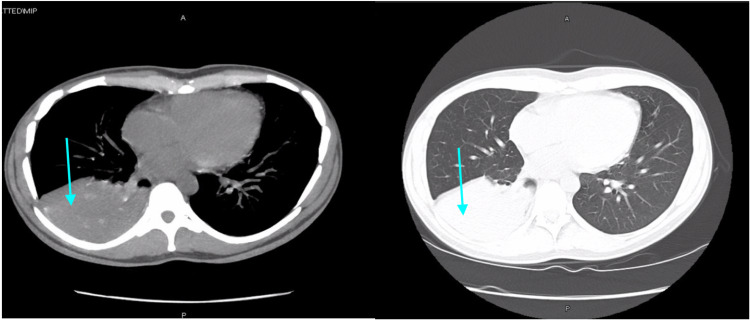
CT chest showing right lower lobe consolidation (blue arrows)
